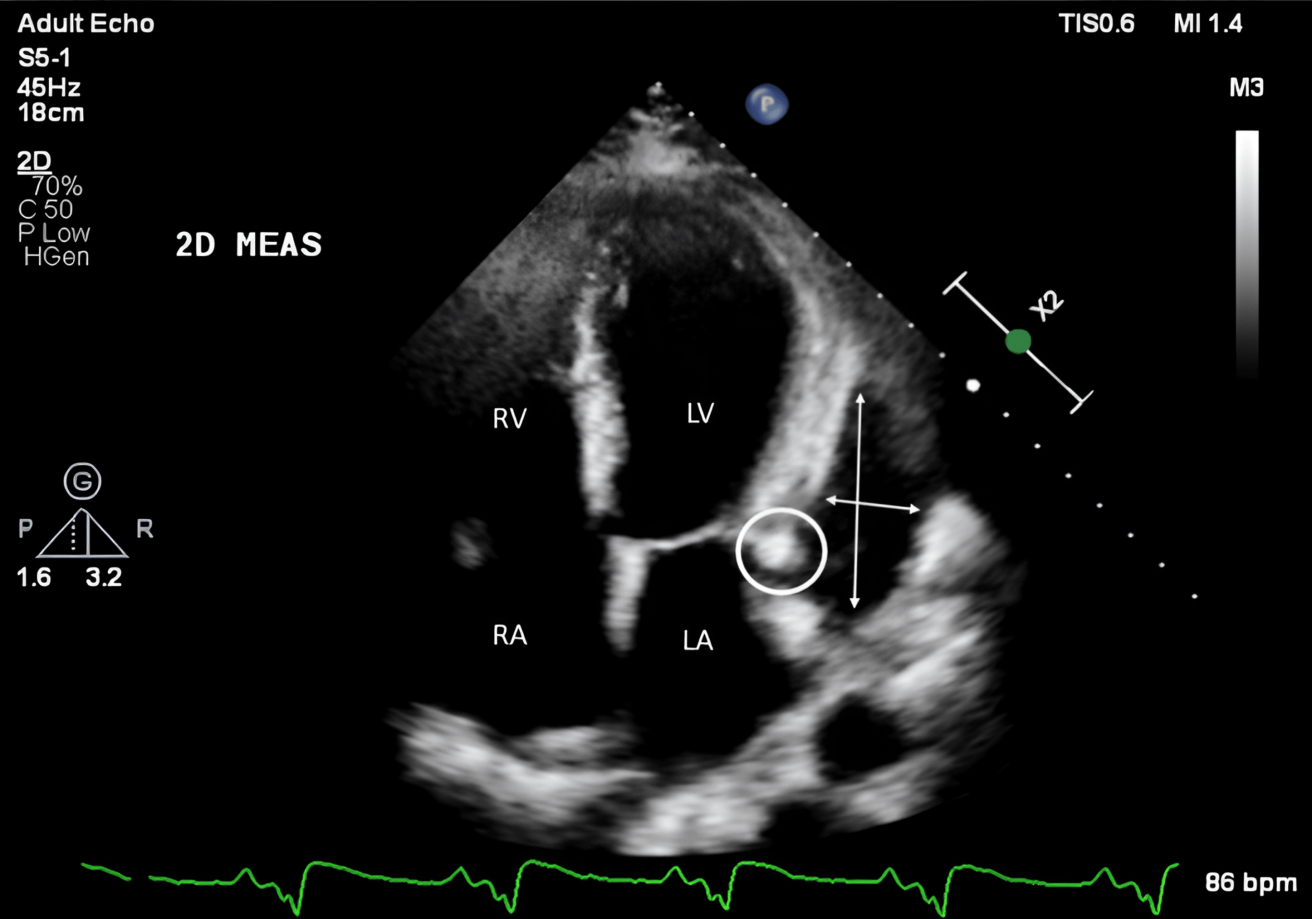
Transthoracic echocardiography suggestive of a hyperechoic structure in the left atrioventricular groove (white circle) and a localized pericardial effusion (white arrows) RA: right atria; RV: right ventricle; LA: left atria; LV: left ventricle
